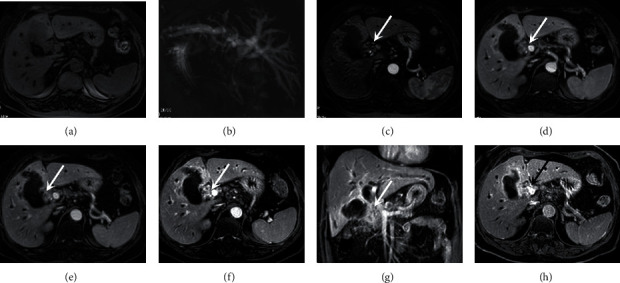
MRI LAVA dynamic enhanced scanning showed clear hilar features. A 72-year-old female patient presented with hilar infiltrating cholangiocarcinoma. The patient had good breath-holding coordination, clear images at all stages, and no noticeable respiratory artifacts. Plain scan showed irregular thickening of the bile duct wall (a), MRCP image showed significant dilation of the intrahepatic bile duct (b), and images of LAVA dynamic enhancement showed irregular thickening of the hilar bile duct wall with “progressive enhancement” (c–h, arrow).
